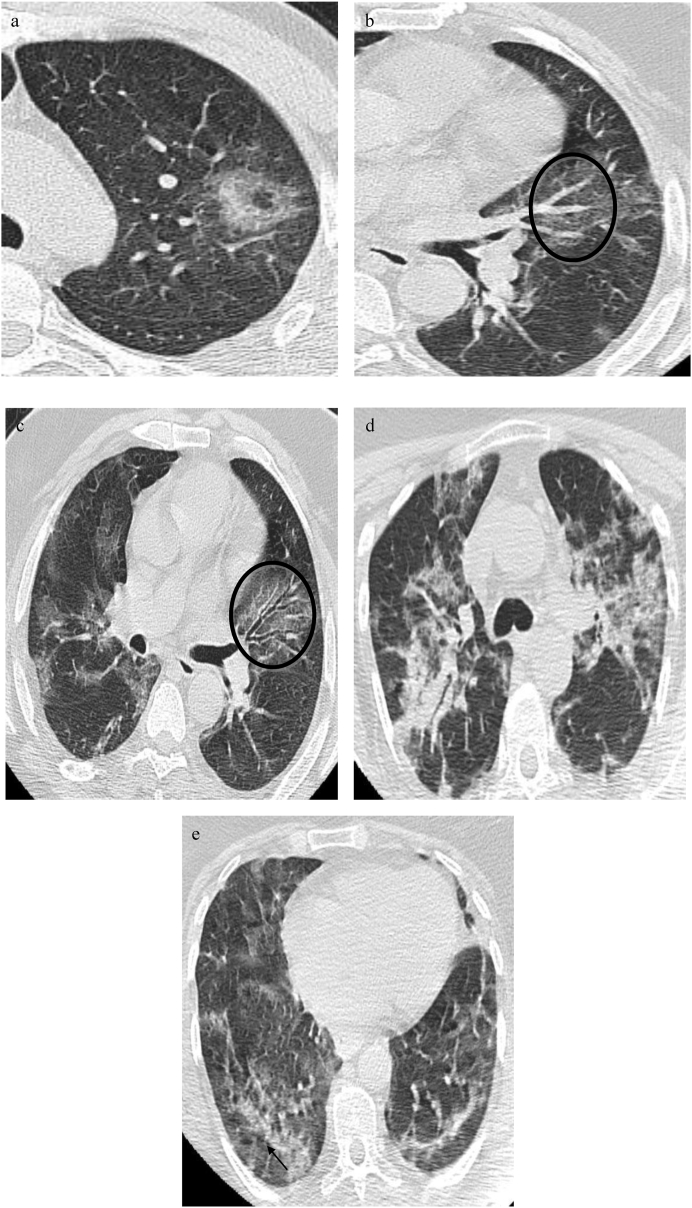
Covid-19 imaging features in patients with a positive RT-PCR at different disease stages. Unenhanced axial CT images of the lung show.*(a) A left upper lobe unifocal rounded ground glass opacity;(b) Patchy peripheral ground glass opacity with vascular dilatation (Black circle);(c) Multifocal, bilateral subpleural ground glass opacities with traction bronchiectasis (Black circle);(d) Extensive bilateral ground glass opacities associated with thickened interlobular and intralobular septa (Crazy paving) alongside with peripheral consolidations;(e) Subpleural band in advanced-phase disease (Black arrow).*
